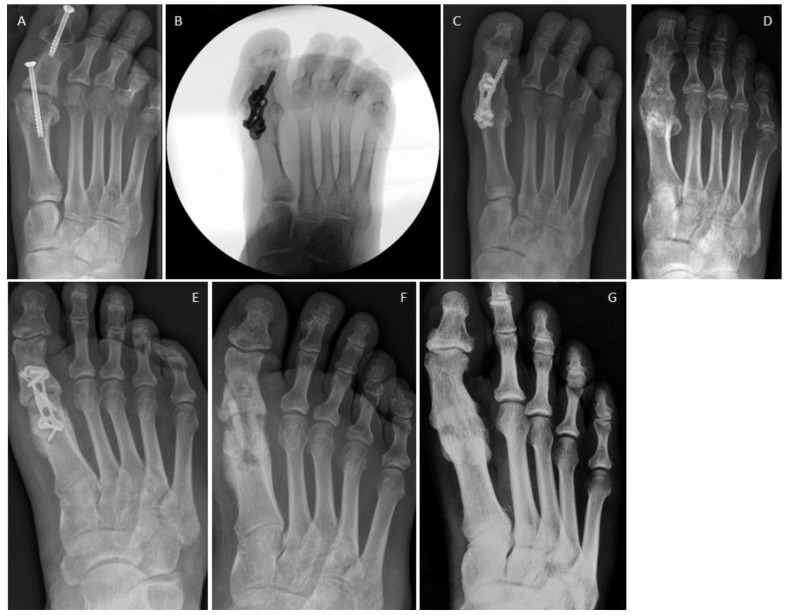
First metatarsophalangeal joints non-unions. (A–D) Conventional revision surgery: (A) Failed arthrodesis at MTP1 (note: the interphalangeal joint was successfully fused); (B) intraoperative fluoroscopy after MTP1 revision surgery with a lag screw and a plate; (C) radiograph taken 4 months post-revision; (D) radiograph captured 12 months after revision and after metal removal, fusion is obvious. Bone healing was recorded after 8 weeks. (E–G) Revision surgery with human allogeneic cortical bone screws: (E) pre-operative radiograph; (F) post-operative radiograph, with two Shark Screws^®^ used for revision; (G) radiograph taken 12 months post-revision, where the Shark Screws^®^ were hardly visible. Bone healing was recorded after 12 weeks.
